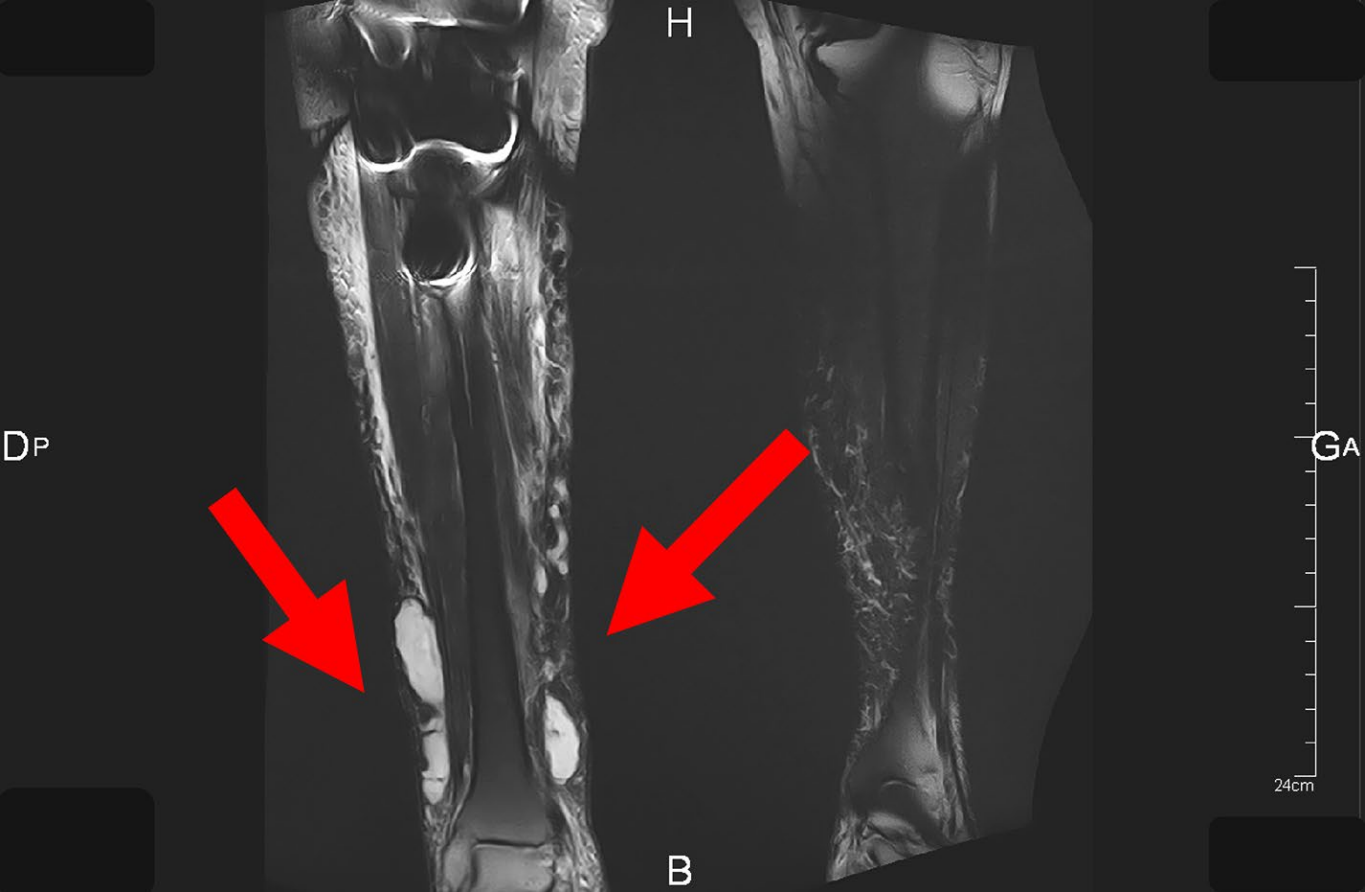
Magnetic Resonance Imaging of the legs. Coronal T2 FAT-SAT (Fat suppression) MRI sequences of the legs, showing several collections in the soft tissues of the right leg (*arrows*).
